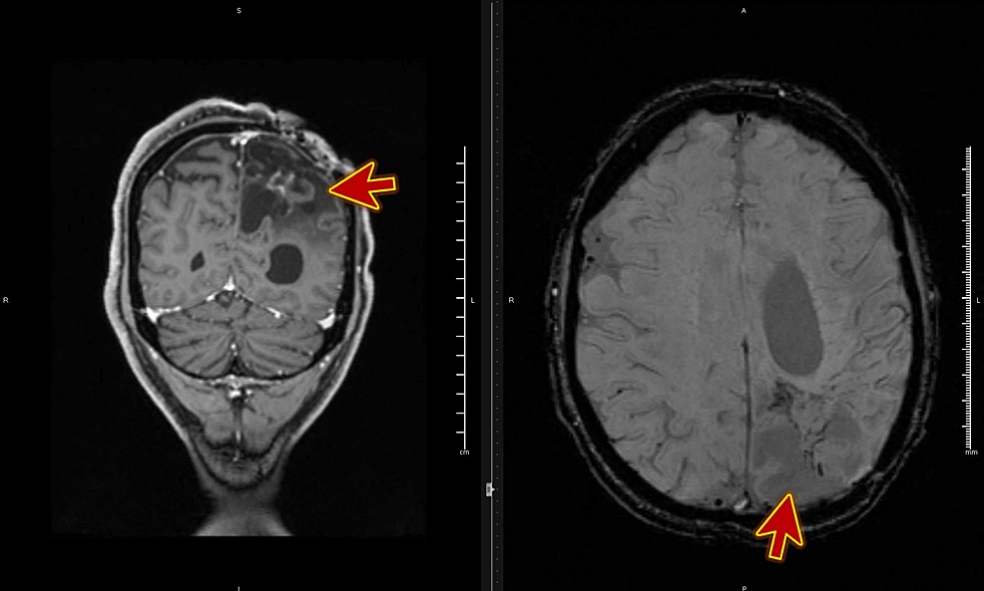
MRI scan of the head with contrast showing postoperative images of glioblastoma multiforme.
